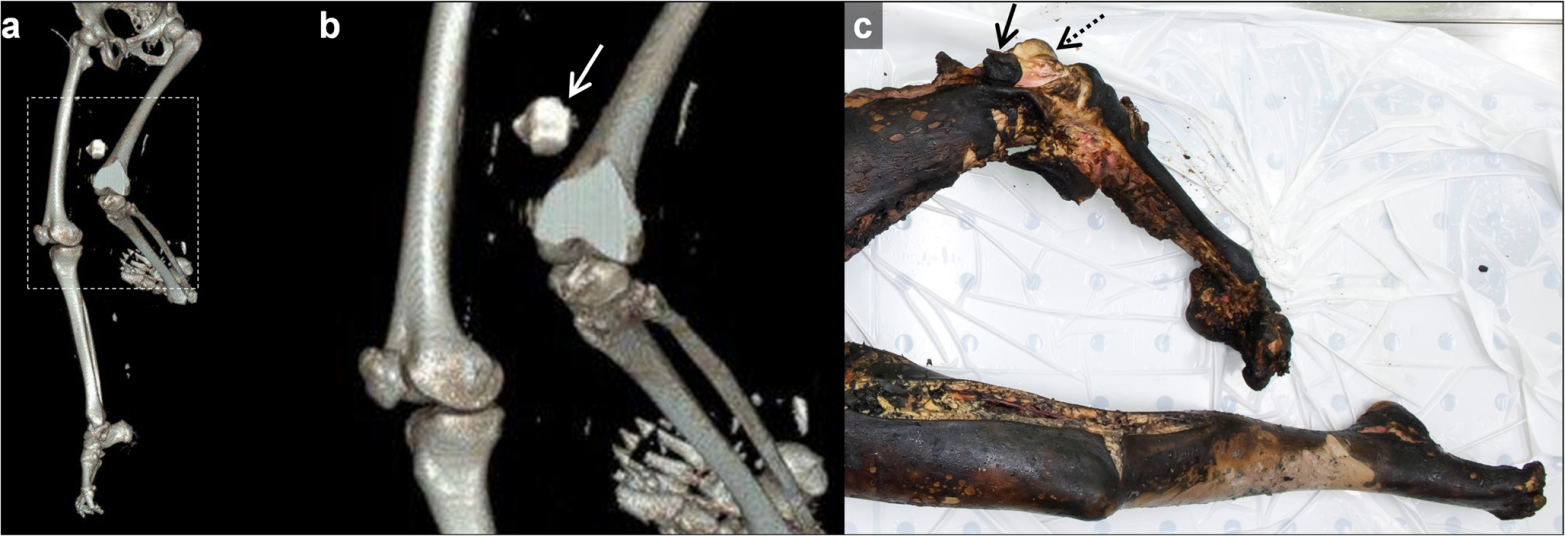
Typical proximal displacement of the patella in the left knee exposed to fire. a Anteroposterior 3D reconstruction of a CT of the lower extremities of a body exposed to fire, presenting patellar displacement. b Enlarged part of the 3D-CT scan in (a). Note the proximal displacement of the patella of the left knee (*arrow*). The patellar ligament is burned through or ruptured by the force of the shrinking rectus femoris muscle. c The proximally displaced and blackened left patella (*black arrow*) could be identified at autopsy. Its normal location is indicated by the *dashed arrow*. Typical flexing of the joints in legs and feet that were exposed to fire can be appreciated. Note also the coverage of the right patella by soft tissues, which prevents the patella from proximal displacement
